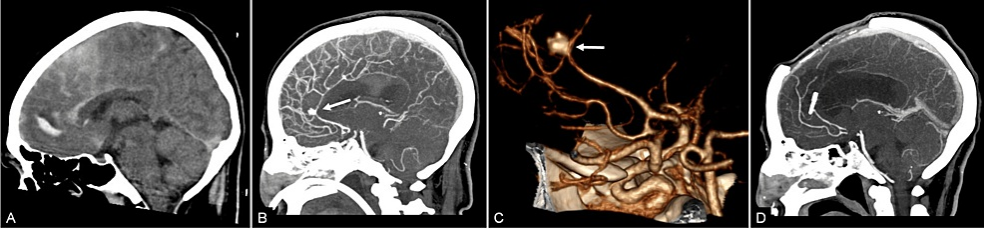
Pre- and postoperative radiological studies of case 5. (A) CT sagittal section showing hyperdense images in the medial surface of frontal lobes and pericallosal cistern. (B) Preoperative CTA sagittal view and (C) 3D reconstruction demonstrating a multilobulated azygos aneurysm (arrow). (D) Postoperative CTA sagittal section reveals frontal craniectomy and exclusion of the aneurysm.
